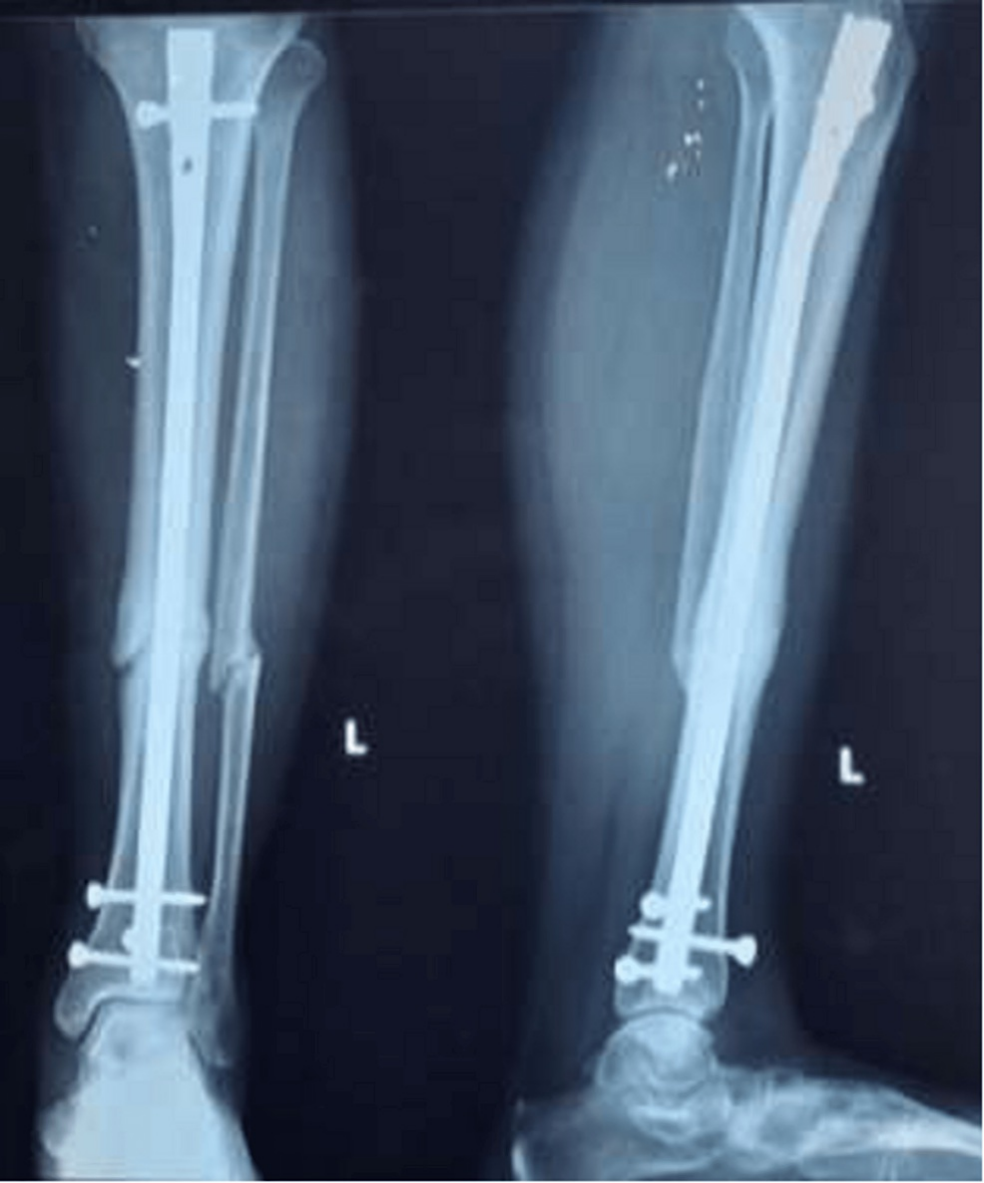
Six-month follow-up radiograph
